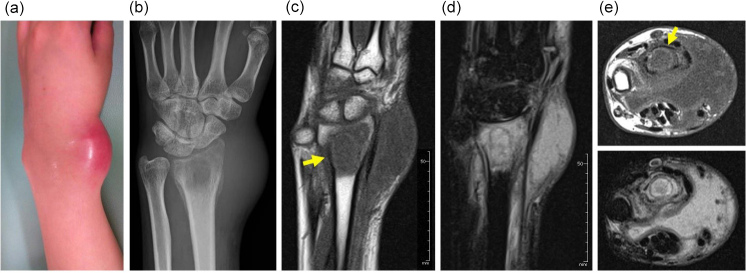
Swelling and redness are seen at the wrist (a). Plain radiography shows an osteolytic lesion in the distal radius with soft tissue swelling (b). Magnetic resonance imaging shows low signal intensity on T1-weighted
